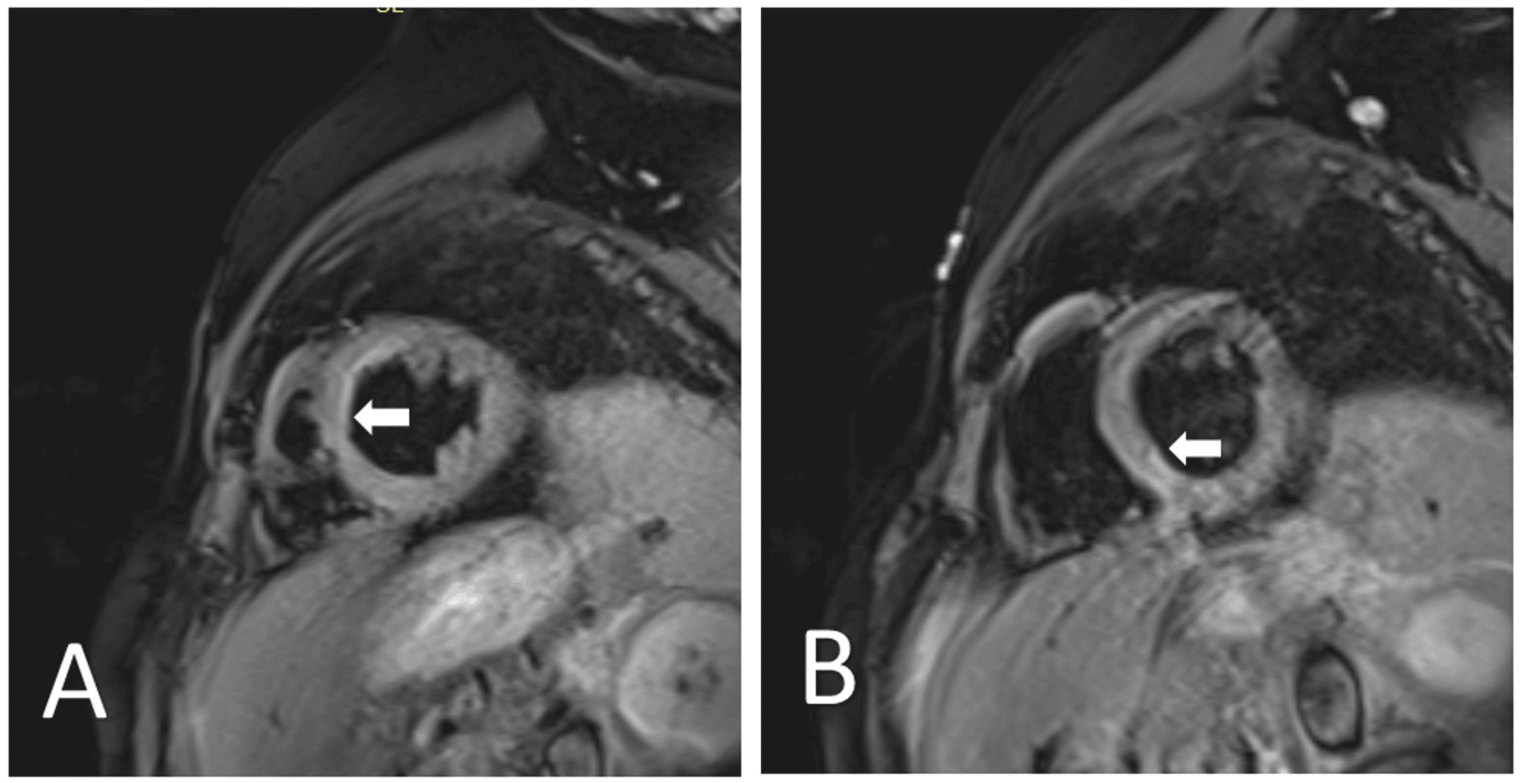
Cardiac MRI (short-axis view, late gadolinium enhancement sequence) The annotated areas in (A and B) demonstrate patchy areas of midwall to subepicardial enhancement predominantly involving the lateral wall of the left ventricle. There is no subendocardial or transmural enhancement in the coronary artery distribution. These findings are non-ischemic in pattern and are highly suggestive of myocarditis.
